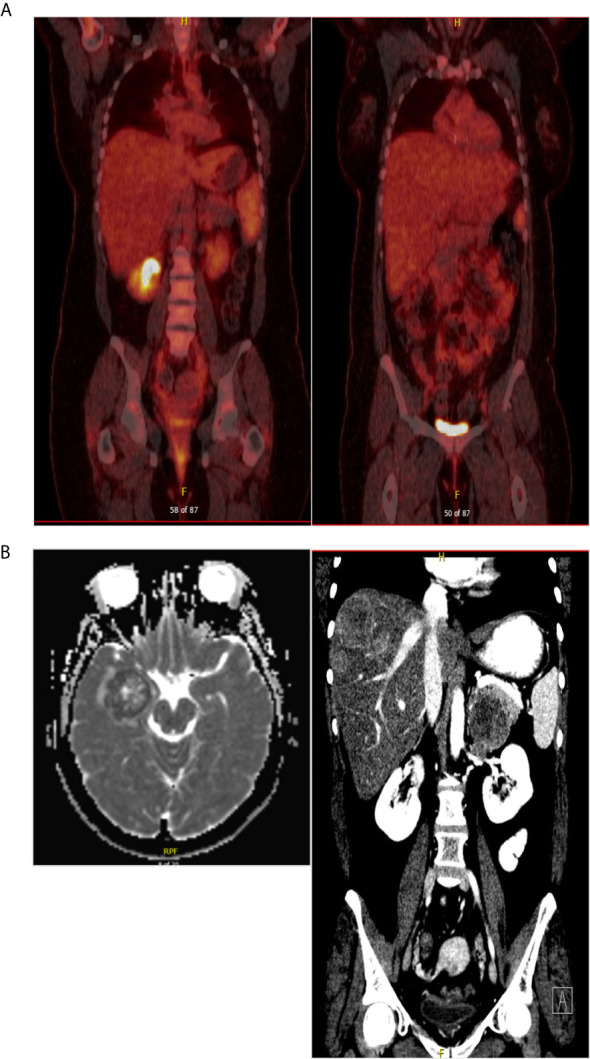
(A) PET/CT scan after completing chemoradiation therapy showed no evidence of disease. (B) At the time of diagnosis of recurrent disease, brain MRI and CT scan of the abdomen confirming brain metastasis and multiple hepatic metastatic disease.
